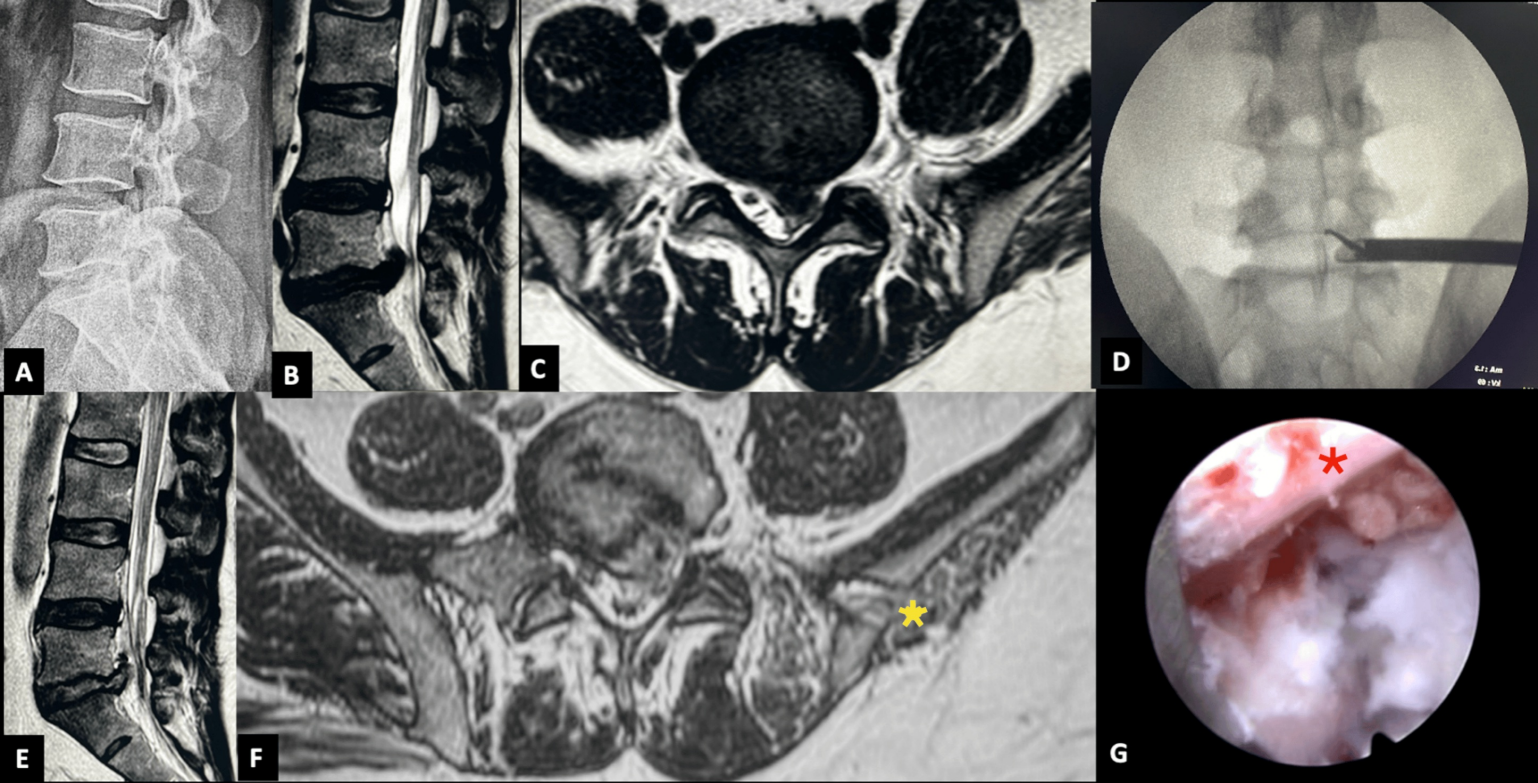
Transiliac transforaminal endoscopic lumbar discectomy in a patient with up-migrated lumbar disc herniation. (A) Lateral radiograph of a 41-year-old male with a high iliac crest. (B, C) MRI showing an up-migrated big disc fragment compressing the central dura and the left S1 root. (D) Per-operative C-arm image of the transiliac approach shows the horizontally positioned endoscope assembly and the hook used to tease the up-migrating fragment. (E) Postoperative MRI showing adequate decompression. (F) Oblique MRI showing the transiliac channel (yellow asterisk) and adequate decompression. (G) Adequacy of decompression with the decompressed pulsatile traversing nerve root (red asterisk).
